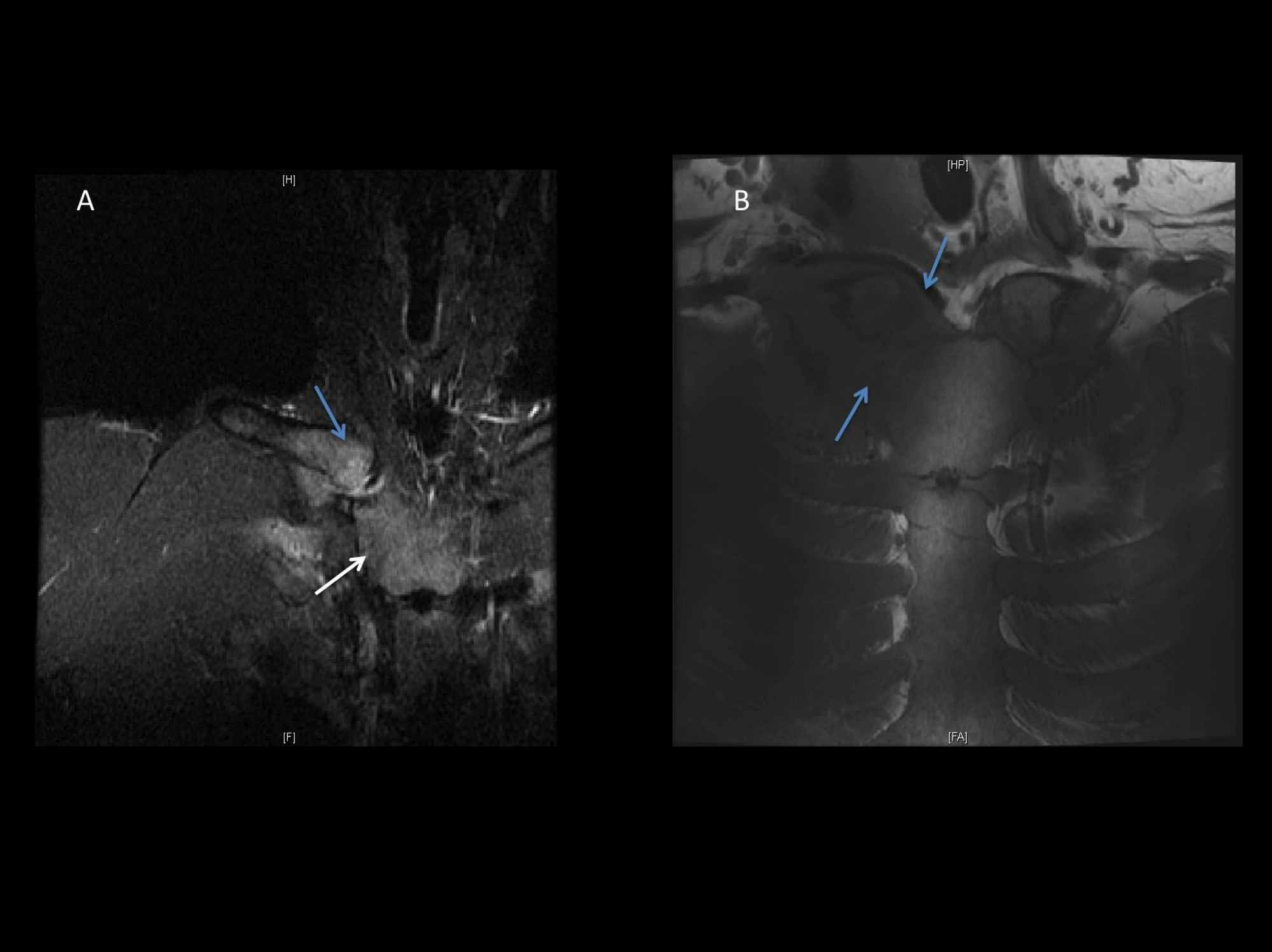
Follow-up MRI of the chest and right SCJ with and without contrast. A: MRI chest and right SCJ with contrast showing right sternoclavicular septic arthritis (blue arrow) with manubrium osteomyelitis (white arrow). B: MRI chest and right SCJ without contrast showing inflammatory changes around the right SCJ. SCJ, sternoclavicular joint
